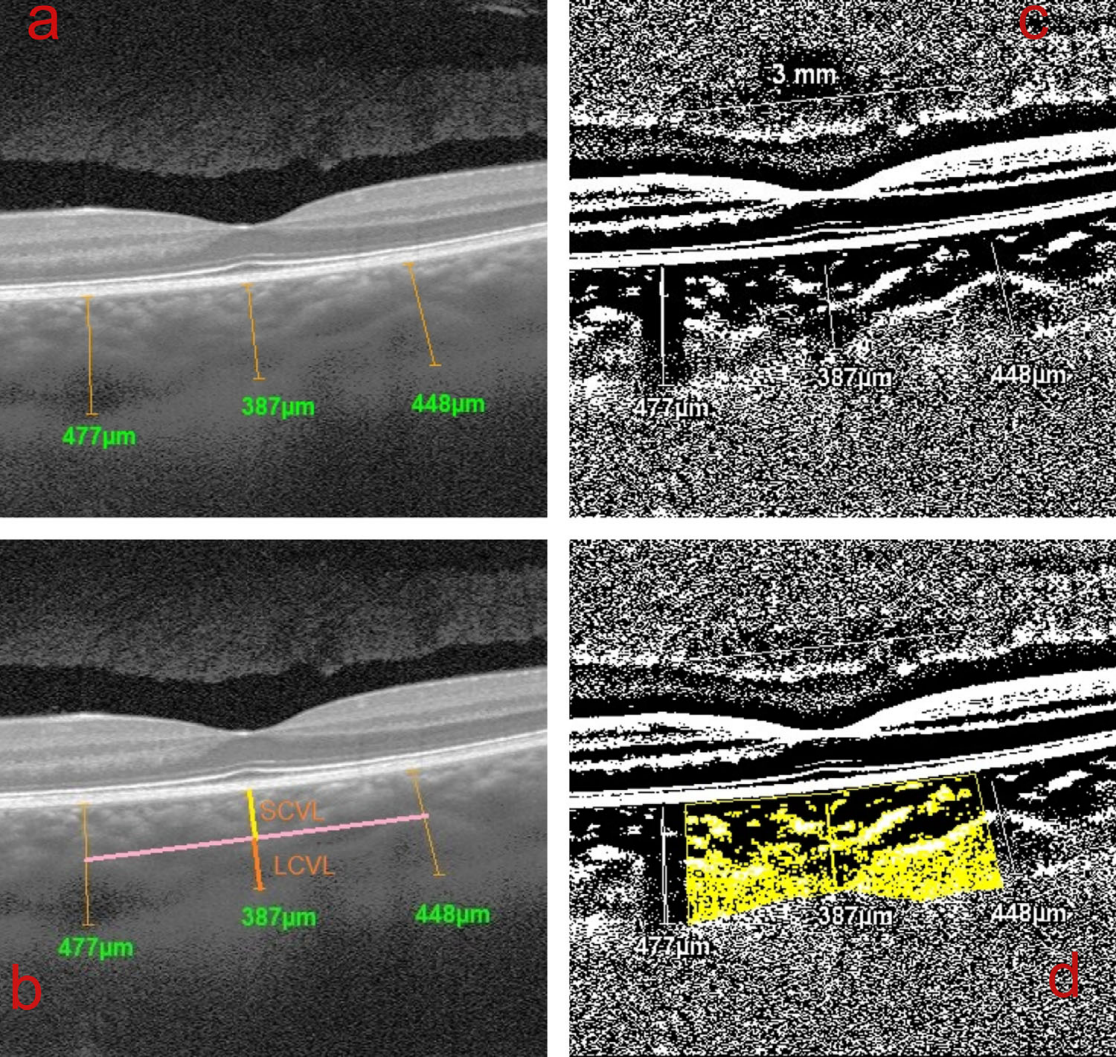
Choroidal thickness (CT) measurement techniques in a sample case. (a) Measurement of total choroidal thickness from 3 points; subfoveal, nasal 1000 µm from center of fovea, 1000 µm temporal from center of fovea. (b) LCVL thickness (Haller's layer) was measured from the inner border of the choroid-scleral junction to the innermost point of the large choroidal vessel at subfoveal location (orange line). Double asterisks indicate large choroidal vessels. Sattler's layer/choriocapillaris layer (SF-SCVL) is the distance from the outer edge of the hyper-reflective retinal pigment epithelium to the orange line and is indicated by the yellow-colored line. Sattler's layer/choriocapillaris layer (SF-SCVL) is separated from the Haller's layer by a purple line. (c) Converted binary image using Image J with the area of interest in the choroid demarcated with a white line. The choroidal area was measured at approximately 3000 µm wide with the margins of 1500 µm nasal and 1500 µm temporal from the foveal center. (d) The color threshold tool is used to select the dark pixels, representing the luminal area. The choroidal vascularity index is computed dividing luminal area by total choroidal area.
